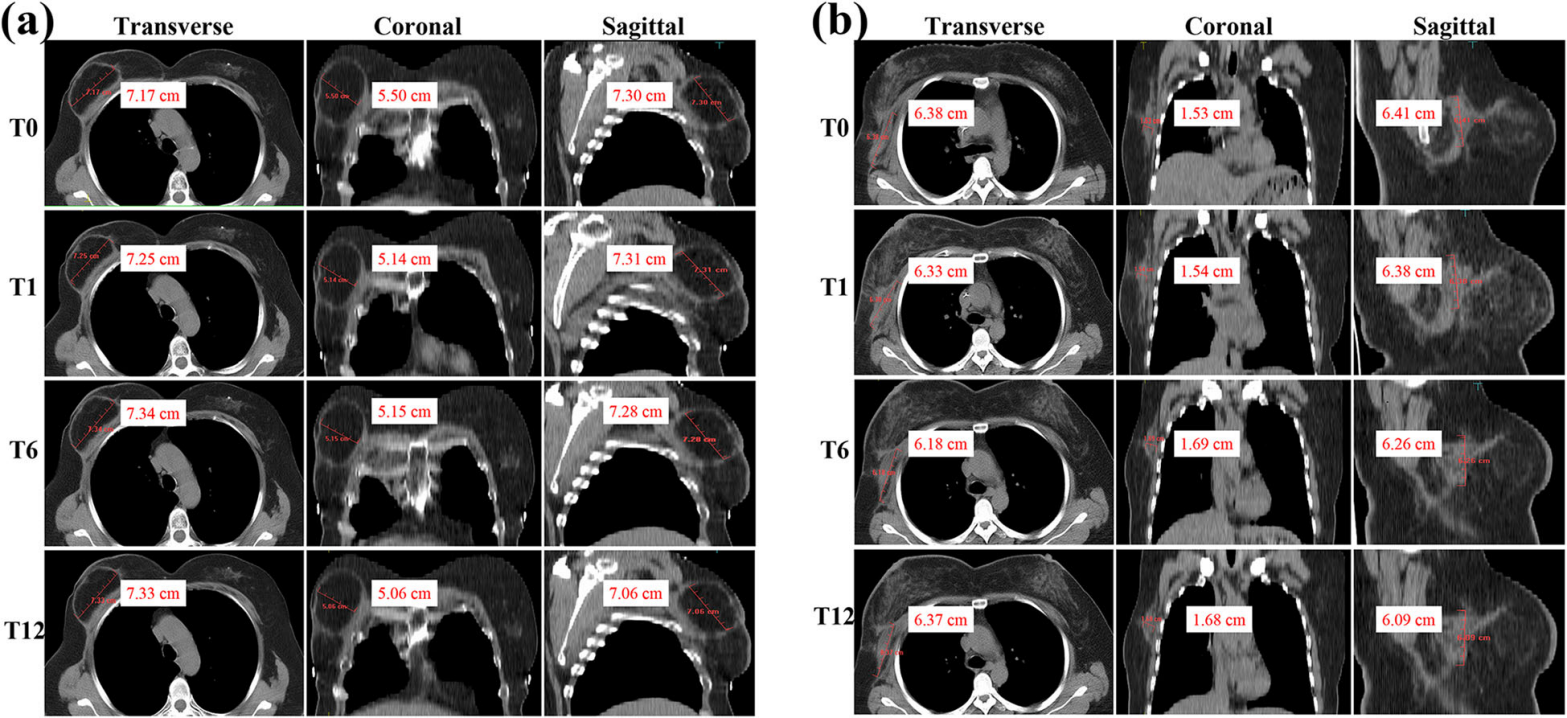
Breast CT scans of two patients who underwent IABR with FDFG (a) and LD flap (b), respectively. The maximum length (left), maximum width (center) and maximum thickness (right) of the flap were measured based on three-dimensional CT scans at T0 (pre-RT), T1 (1 month post-RT), T6 (6 months post-RT), and T12 (12 months post-RT). CT, computed tomography; IABR, immediate autologous breast reconstruction; FDFG, free dermal fat graft; LD, latissimus dorsi
